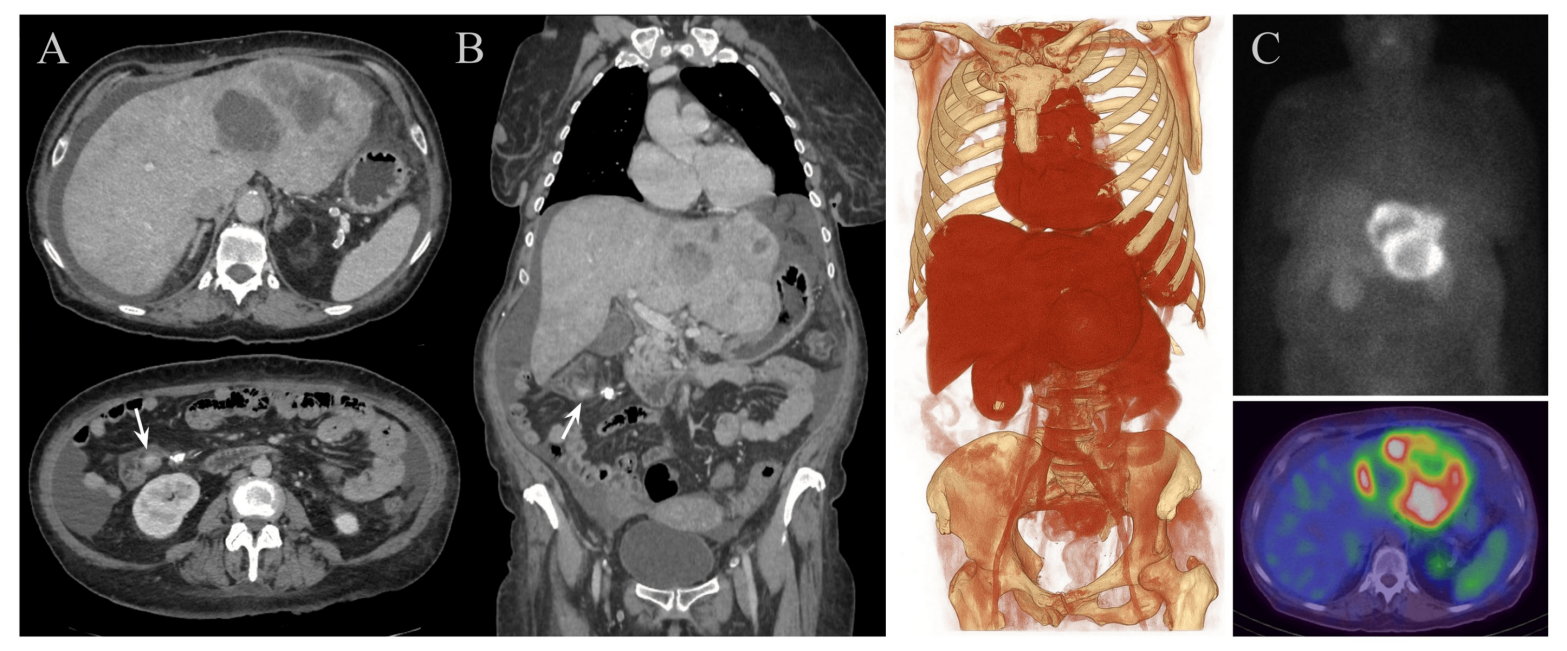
Computed tomography (CT) and single-photon emission computed tomography (SPECT) images of the metastatic tumor. (A-B) Contrast-enhanced CT images in the portal phase shown in axial and coronal planes, respectively, and a 3D volumetric reconstruction demonstrating two large metastases with necrotic centers in the left hepatic lobe, measuring 88 and 94 mm, respectively; mild ascites; and a hyperenhancing nodular lesion (arrow) of approximately 12 mm arising from the terminal ileum near the ileocecal valve, with an adjacent calcified lymphadenopathy and a circumferential mesenteric desmoplastic reaction (“wheel-spoke” pattern), highly suggestive of a metastatic intestinal carcinoid tumor. (C) SPECT with technetium-99m-hydrazinonicotinyl-Tyr³-octreotide (⁹⁹mTc-HYNIC-TOC) demonstrating somatostatin receptor expression at the margins of the hepatic metastases, visible both in volumetric reconstructions and on fused CT axial images.
